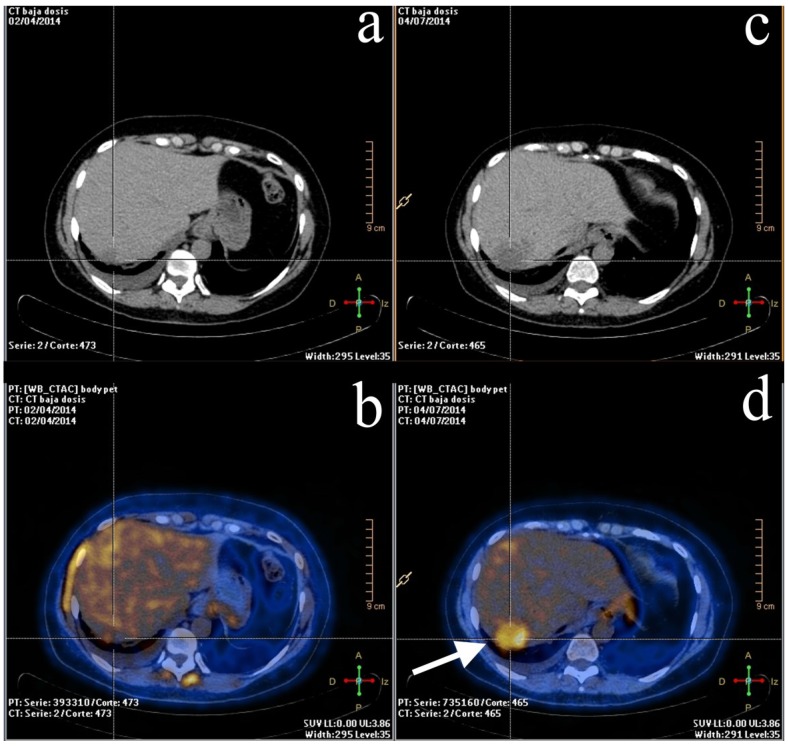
A 53-year-old woman with breast cancer in the course of therapy but with rising biomarkers. The patient was referred for FDG-PET/CT evaluation. *Left column*: baseline PET/CT scan. (a) Non-enhanced CT showing a hypodense lesion in the right posterior hepatic lobe; (b) PET/CT. Mild metabolism was shown in the lesion, similar to what was found in the background. *Right column*: mid-therapy PET/CT scan. (c) Non-enhanced CT showing the same lesion but significantly larger than baseline exam. (d) Hypermetabolic lesion with heterogeneous distribution of the radiotracer (arrow) shown on PET/CT, consistent with metastatic liver lesion.
